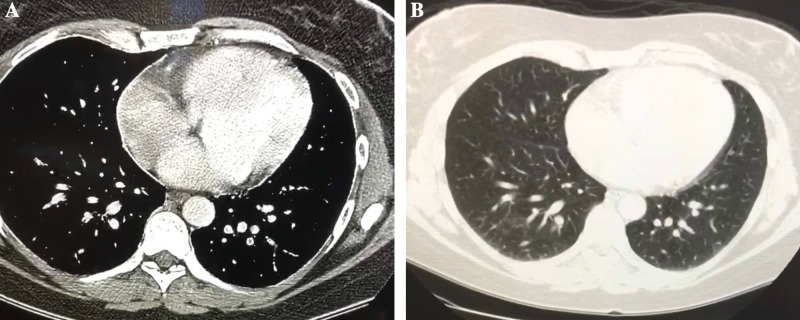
Computed tomography (CT) scans. A: Chest CT with contrast enhancement in the transverse plane, B: Chest CT without contrast enhancement in the transverse plane.
